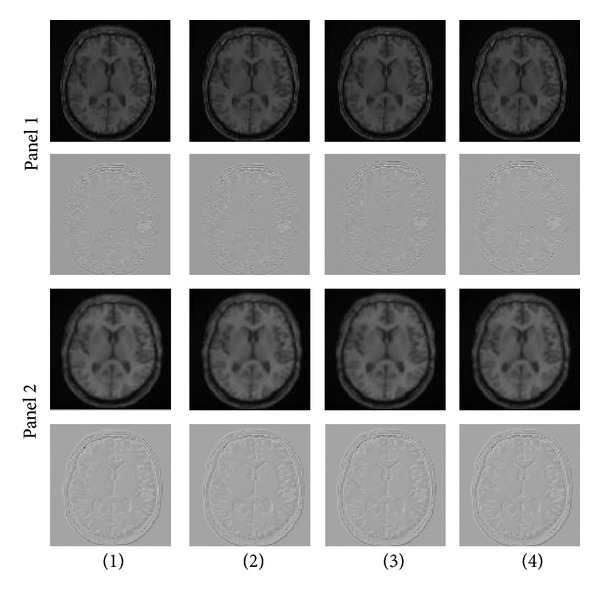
In the top panel one, axial slices from two unregistered 3D MRI volumes using an intensity-based cost function (CR (first column), LS (second column), NCC (third column), and NMI (fourth column)) are shown. Windowed Sinc interpolation was used during registration for the panel one. The second row of the panel one shows subtraction of the images (registered and reference images). The panel two shows similar axial slices from the same two data sets after registration of the full 3D volumes using the same intensity-based cost function (CR (first column), LS (second column), NCC (third column), and NMI (fourth column)). B-spline 3rd-order interpolation was used during registration for the panel two. The second row of the panel two shows subtraction of the images. Although differences of the first rows of panel one and panel two are not easily observed by eye, subtraction of the images shows that differences are present, as seen on the second rows of panel one and panel two.
